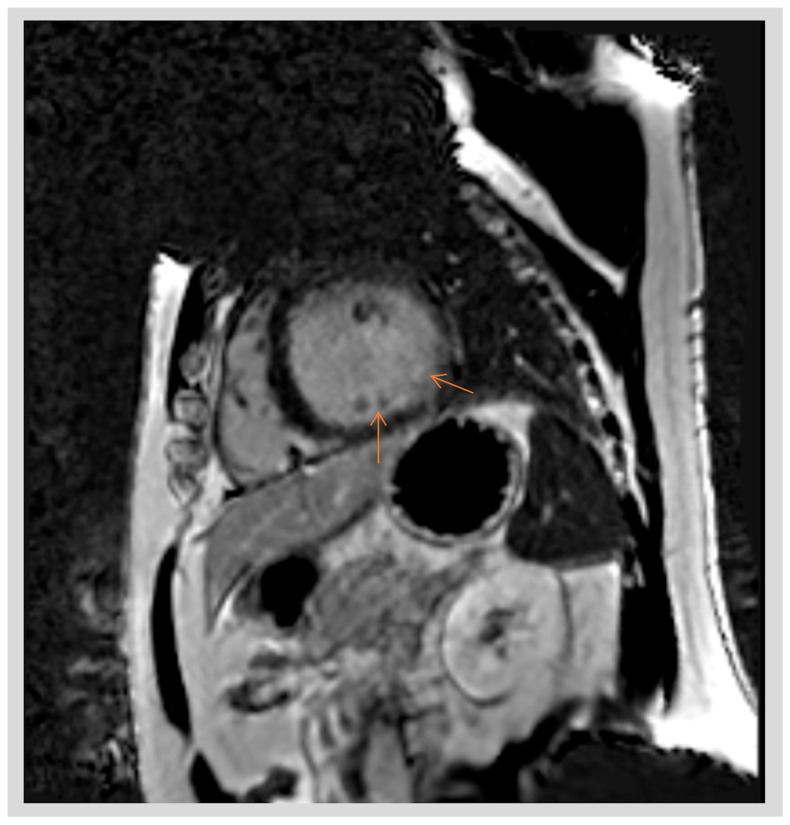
Cardiac MRI short-axis view of the left ventricle in a patient with AMVPS demonstrating areas of late gadolinium enhancement (LGE) affecting the posterolateral left ventricle and papillary muscle (arrows).
